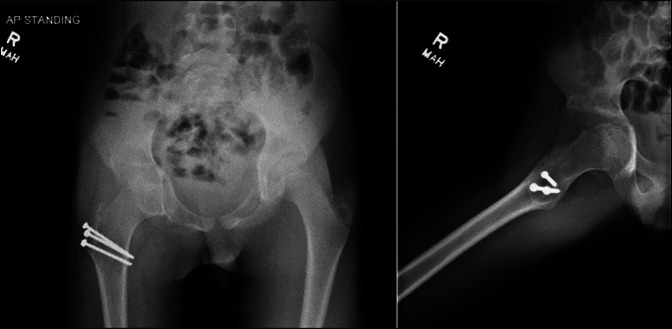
Radiographs at 4 months postoperatively showing right femoral head seated in acetabulum with medialization of hip center, acetabular protrusio relative to contralateral side. No evidence of recurrence or subluxation.
